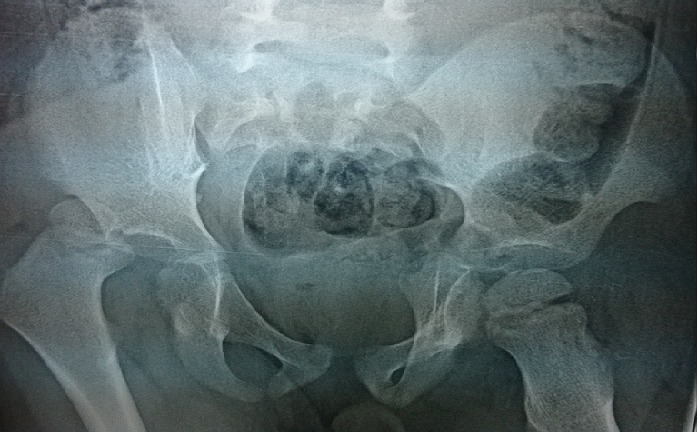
Standard radiography of the pelvis showing an aspect of a high pure right posterior iliac variety dislocation without fracture lesions
